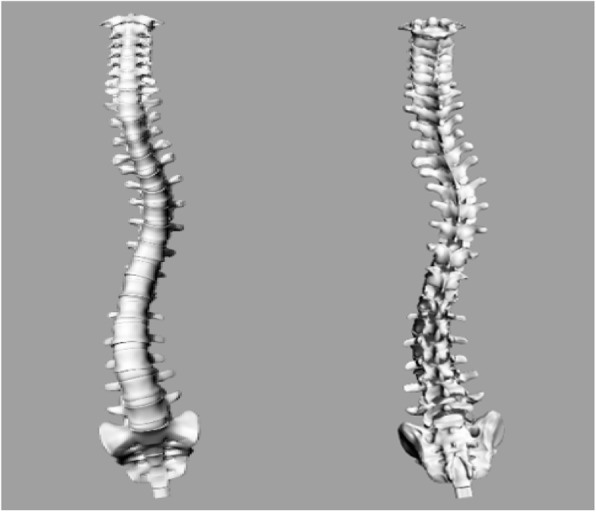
The CT scan for considered vertebral column and its 3D model
